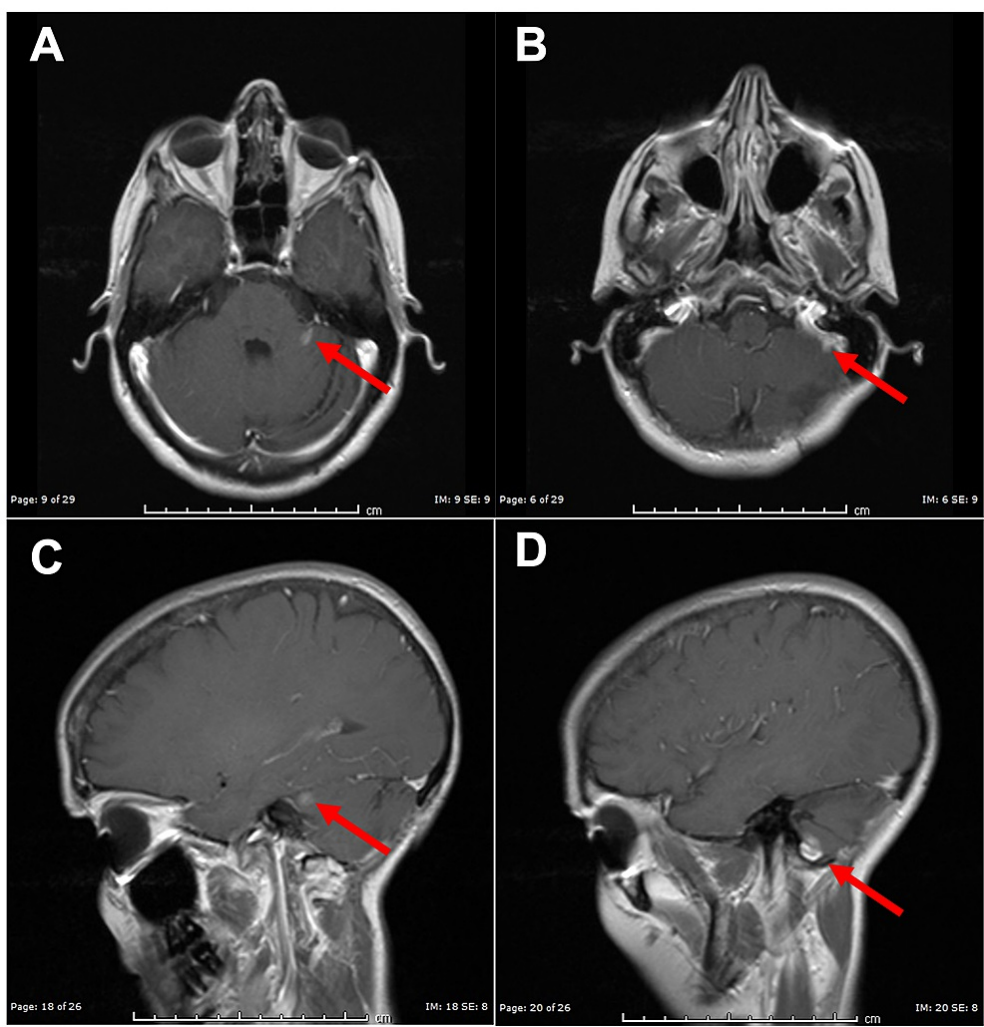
MR Images Following Second Dose of Stereotactic Radiosurgery (A, B) Axial T2-weighted MR images demonstrating improvement in two enhancing lesions within the left cerebellum after SRS. (C, D) Sagittal T1-weighted MR images with gadolinium contrast demonstrating improvement in two enhancing lesions within the left cerebellum after SRS. SRS: stereotactic radiosurgery.
